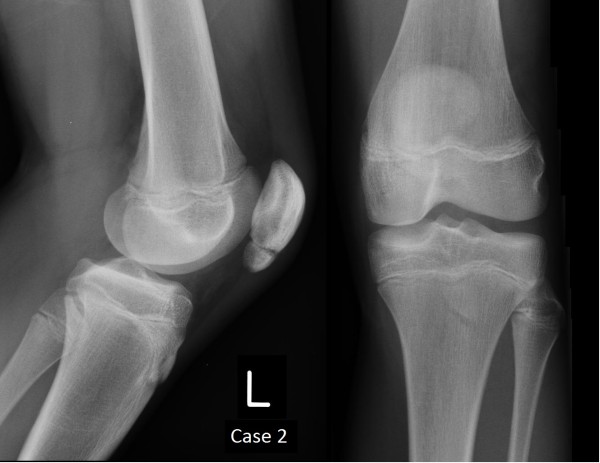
Plain film radiographs of Case 2 at 12 weeks.
